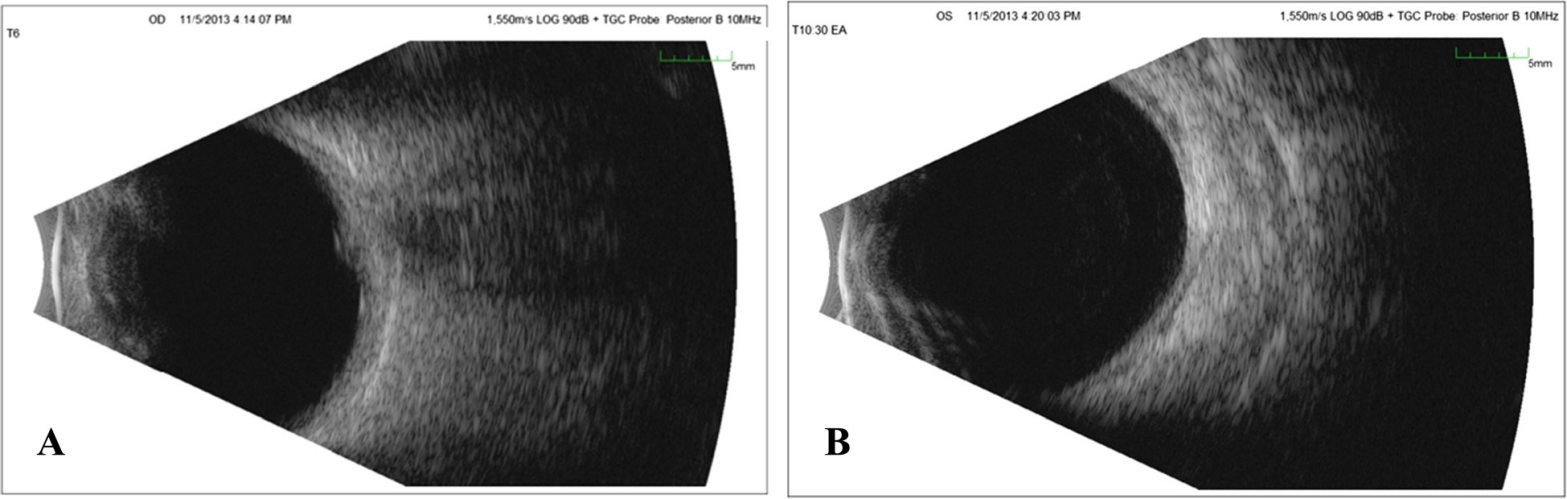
B-scan ultrasound of the right and left eyes. (A) B-scan ultrasound of the right eye: elevation of optic nerve head. (B) B-scan ultrasound of the left eye: no visible elevation could be appreciated in the superonasal quadrant of the left eye.
